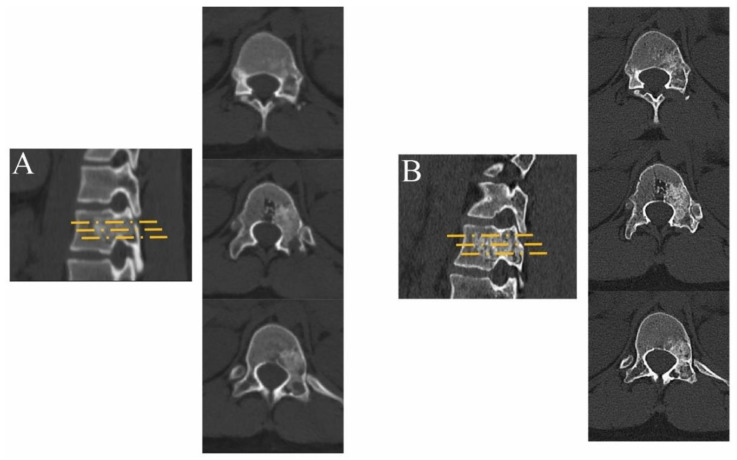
(A) Two days after the operation, CT showed that the lesion was completely cleared and the bone graft was fully filled. (B) Half a year after the operation, CT showed that the lesion area was completely filled with allograft bone, indicating that the bone graft was successful.
